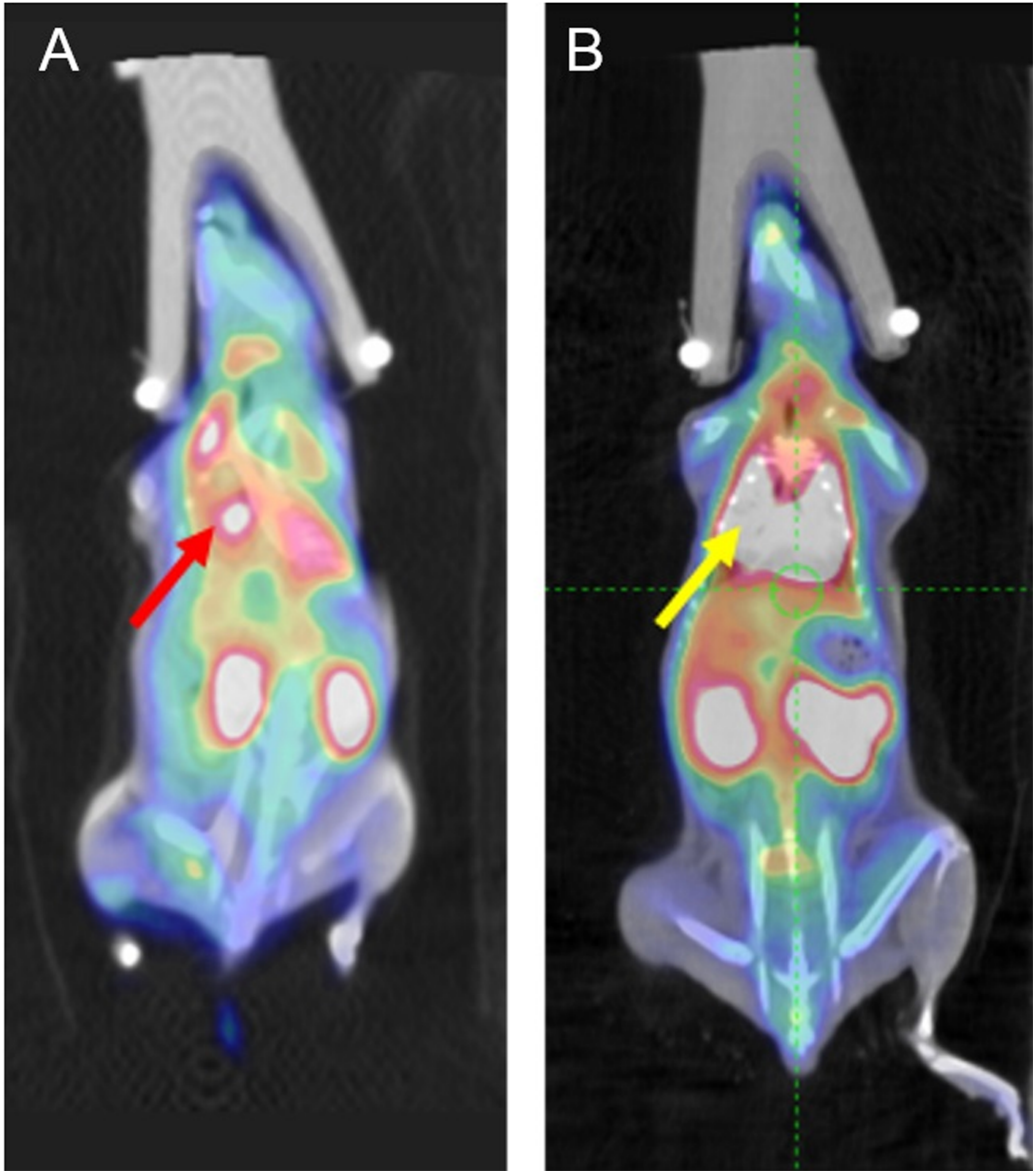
Examples of visual analysis. (A) In situ tumor. Red arrow: high FDG uptake caused by tumor inside the lung (value of SUV was around 1.7) (B) In situ inflammation. Yellow arrow: high FDG uptake caused by inflammation inside the lung (value of SUV was also around 1.7)
